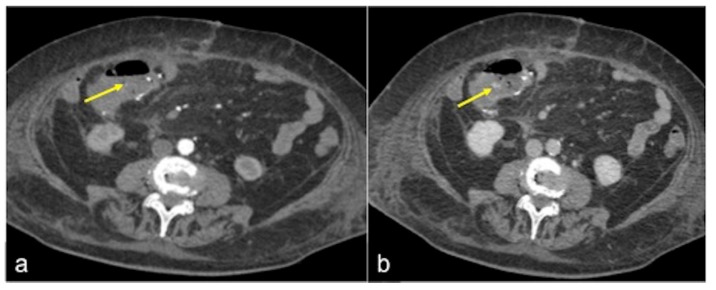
CTA multiphasic study of slow flow bleeding in arterial (a) and venous (b) phases. The haemorrhagic spot at the surgical site of ileo-colic anastomosis appears inconspicuous in the arterial phase ((a) arrow) and is better identifiable in the venous phase ((b) arrow).
